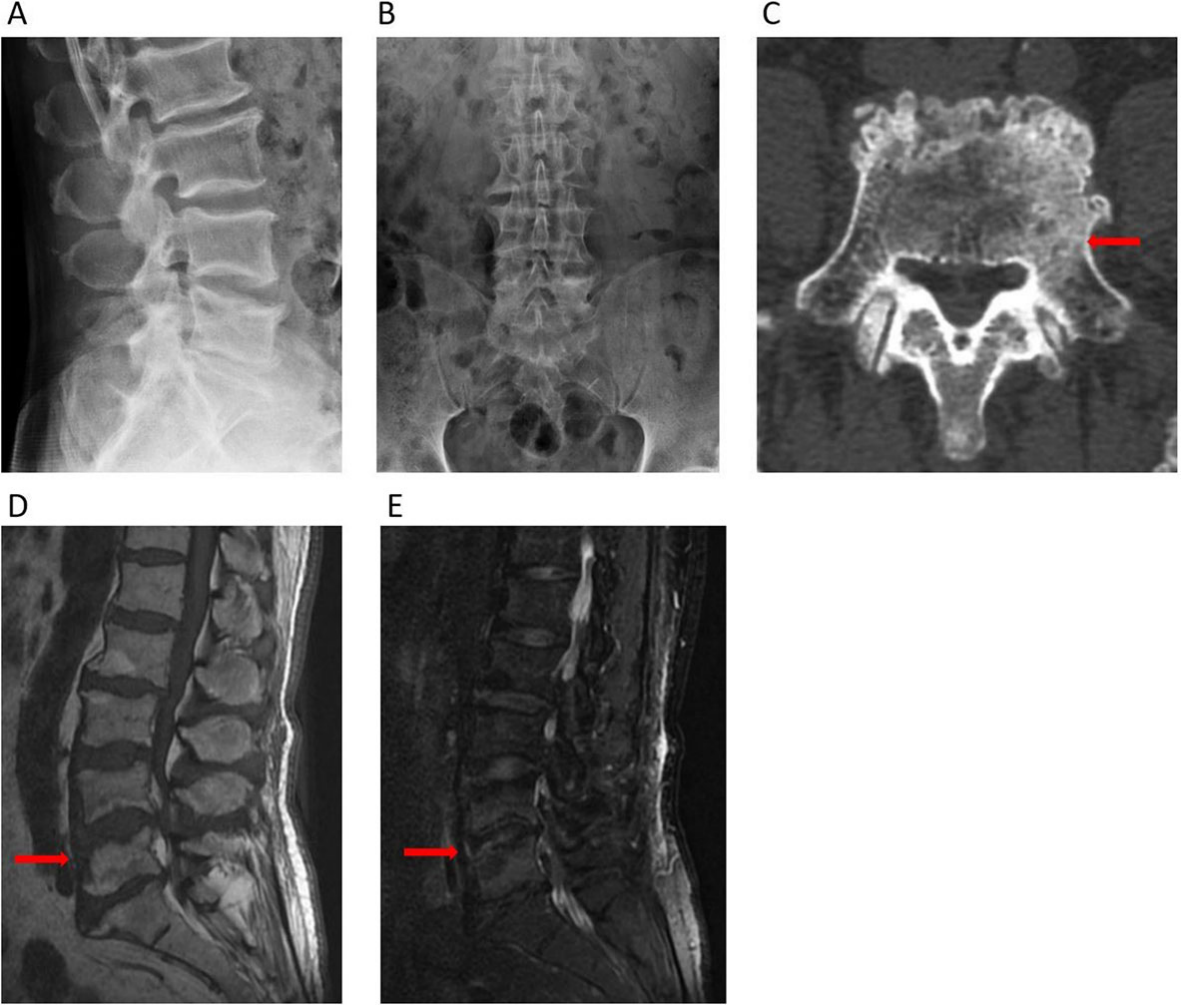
Radiological findings at the 12th month after treatment. Notes: (a and b) X-ray showed degeneration of lumbar spine, hyperplasia of vertebrae, no significant narrowing of L4–5 inter-vertebral space. (c) CT showed the area of vertebral destruction was almost recovered and the bone sclerosis around the lesion (arrow). (d and e) MRI showed the area of inflammatory signal (hypointense on T1-W images and heterogeneous hyperintense on T2-W images) almost disappeared (arrow)
